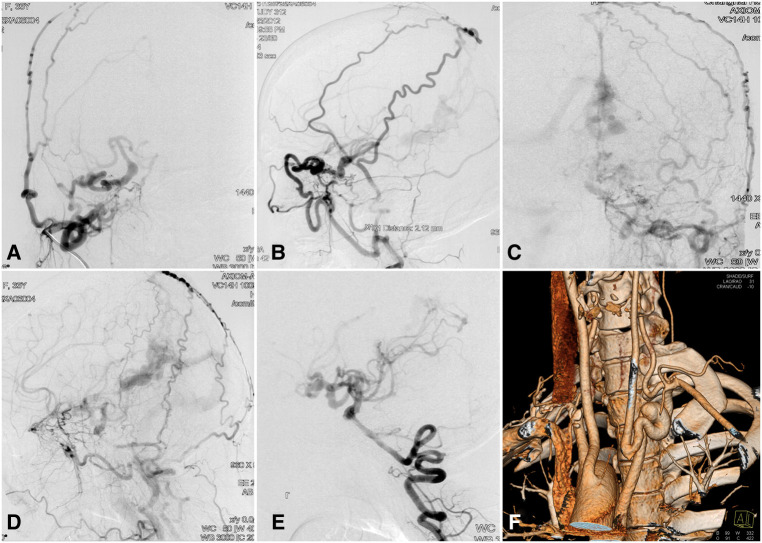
Complicated dural arteriovenous fistula with bilateral ICA agenesis and aortic anomaly in a 33-year-old woman. Contrast injection in the right common carotid artery in (A) lateral and (B) anteroposterior views showing carotid rete mirabile recruiting the anastomotic vessels between the ICA and ECA branches. Contrast injection of the left common carotid artery in (C) lateral and (D) anteroposterior views showing a complicated dural arteriovenous fistula in the region of the straight sinus. (E) Blood supply of the anterior circulation is provided partly by the posterior circulation *via* the posterior communicating artery. (F) CT angiography showing right-sided aortic arch and aberrant left subclavian artery. CT, computed tomography; ECA, external carotid artery; ICA, internal carotid artery.
